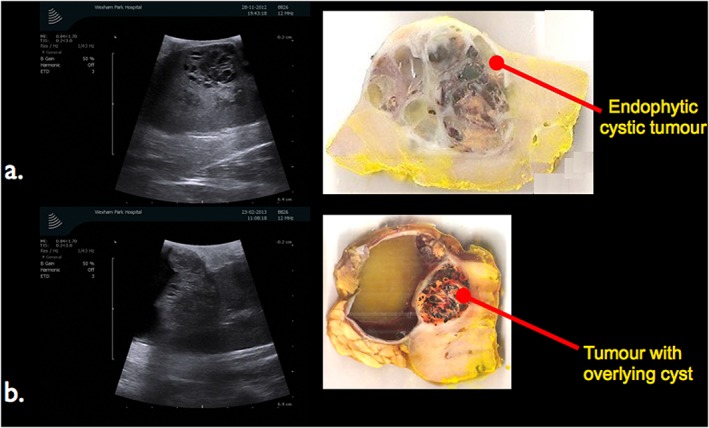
Intra‐operative ultrasound scan imaging of kidney to demonstrate location and nature of an endophytic cystic tumour and a tumour with large overlying cyst. Ultrasound imaging ensures tumour is excised with a small margin of healthy renal tissue
